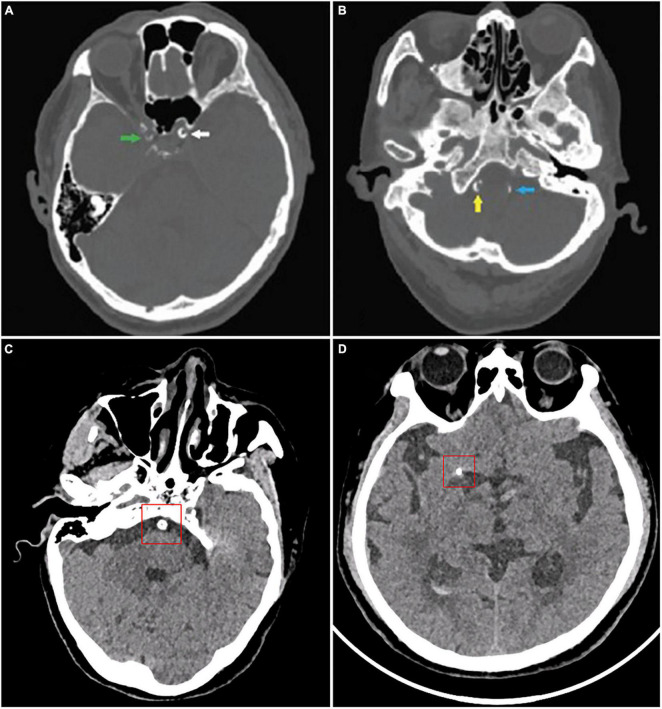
CT images of intracranial artery calcification. According to Babiarz’s method, calcifications were graded as follows: (A) Calcifications in the right internal carotid artery (green), 3 for extent and 2 for thickness; left internal carotid artery (white), 4 for extent and 3 for thickness. (B) Calcifications in the right vertebral artery (yellow), 3 for extent and 3 for thickness; left vertebral artery (blue), 2 for extent and 2 for thickness. According to Woodcock scoring, calcifications were graded as follows: (C) Calcifications in the basilar artery, severe (thick, continuous). (D) Calcifications in the middle cerebral artery calcification, moderate (thick, discontinuous). (A,B) Were from Wu et al. (2016).
